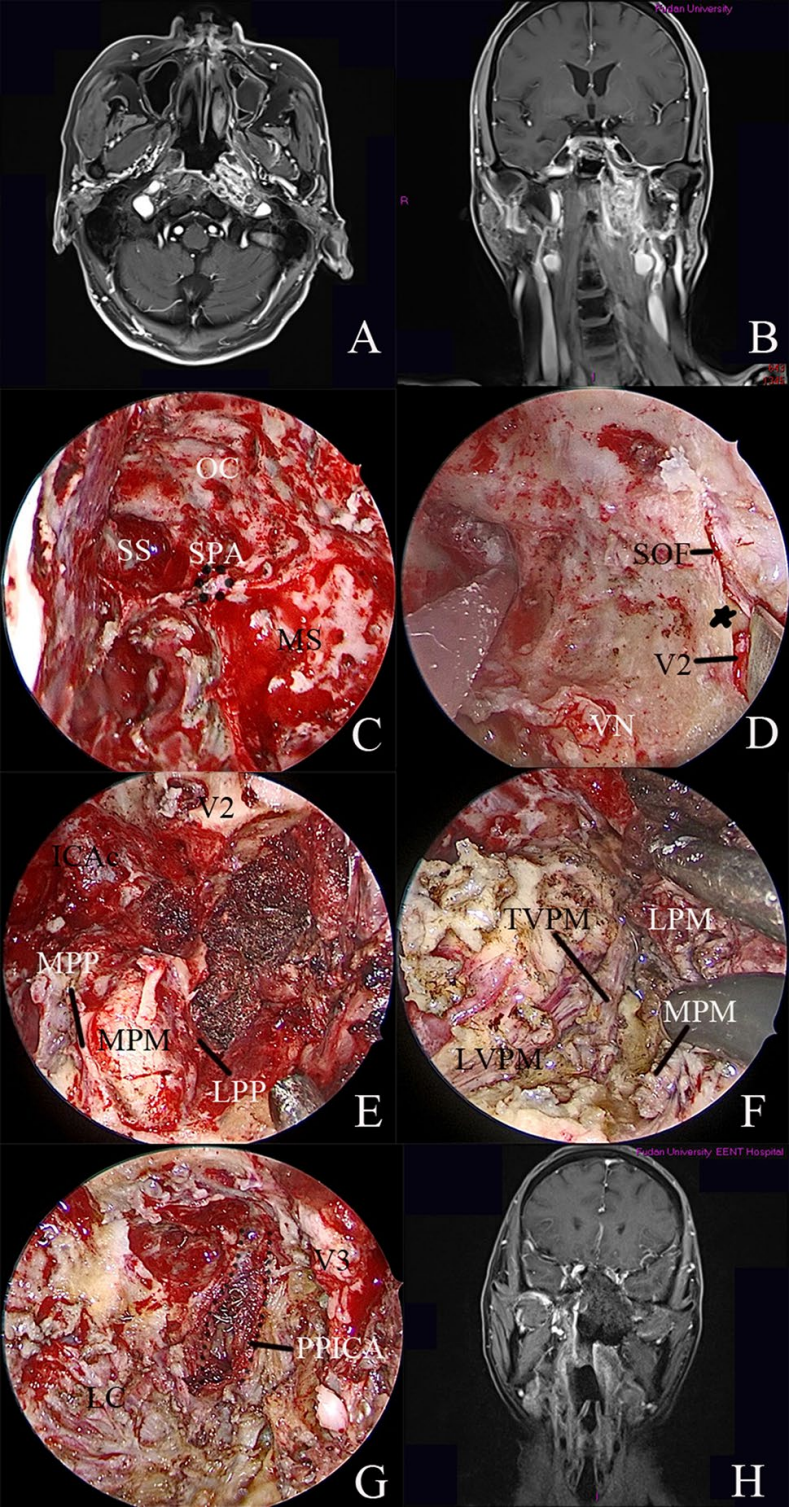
Case 1* Recurrent nasopharyngeal carcinoma. a, b MRI with gadolinium revealed that the mass was located in the left nasopharyngeal fossa, extending into the upper parapharyngeal space with involvement of the posterior wall of the nasopharynx, longus capitis muscle and parapharyngeal and petrous internal carotid arteries. b The internal carotid artery was occluded after the BOT. c Anteroposterior ethmoidectomy, maxillary antrostomy, and sphenoidotomy were completed to expose the guiding landmarks for providing a panoramic view prior to embarking on the approach to the pterygopalatine fossa and the infratemporal fossa. d Endoscopic view of the middle skull base. e, f The upper parapharyngeal space was dissected via the endoscopic transnasal transmaxillary approach. g The intrapetrous and parapharyngeal internal carotid arteries were exposed. h Postoperative MRI indicated complete resection of the recurrent nasopharyngeal carcinoma. *OC* optic canal, *ICAc* paraclival segment of the internal carotid artery, *LC* longus capitis, *LPM* lateral pterygoid muscle, *LPP* lateral pterygoid plate, *LVPM* levator veli palatini muscle, *MPM* medial pterygoid muscle, *MPP* medial pterygoid plate, *PPICA* parapharyngeal internal carotid artery, *SPA* sphenopalatine artery, *SS* sphenoid sinus, *SOF* superior orbital fissure, *TVPM* tensor veli palatini muscle, *VN* vidian nerve, *V2* maxillary nerve, *V3* mandibular nerve, black dotted line, the parapharyngeal internal carotid artery, asterisk: maxillary strut
